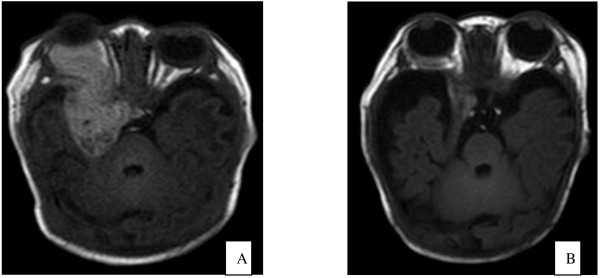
MRI of the intracranial hemangioma in one case with a huge intracranial hemangioma before and after treatment. (A) Exophthalmos of the right eye and a huge intracranial hemangioma. (B) Reduction of exophthalmos of the right eye, and regression of the intracranial hemangioma.
